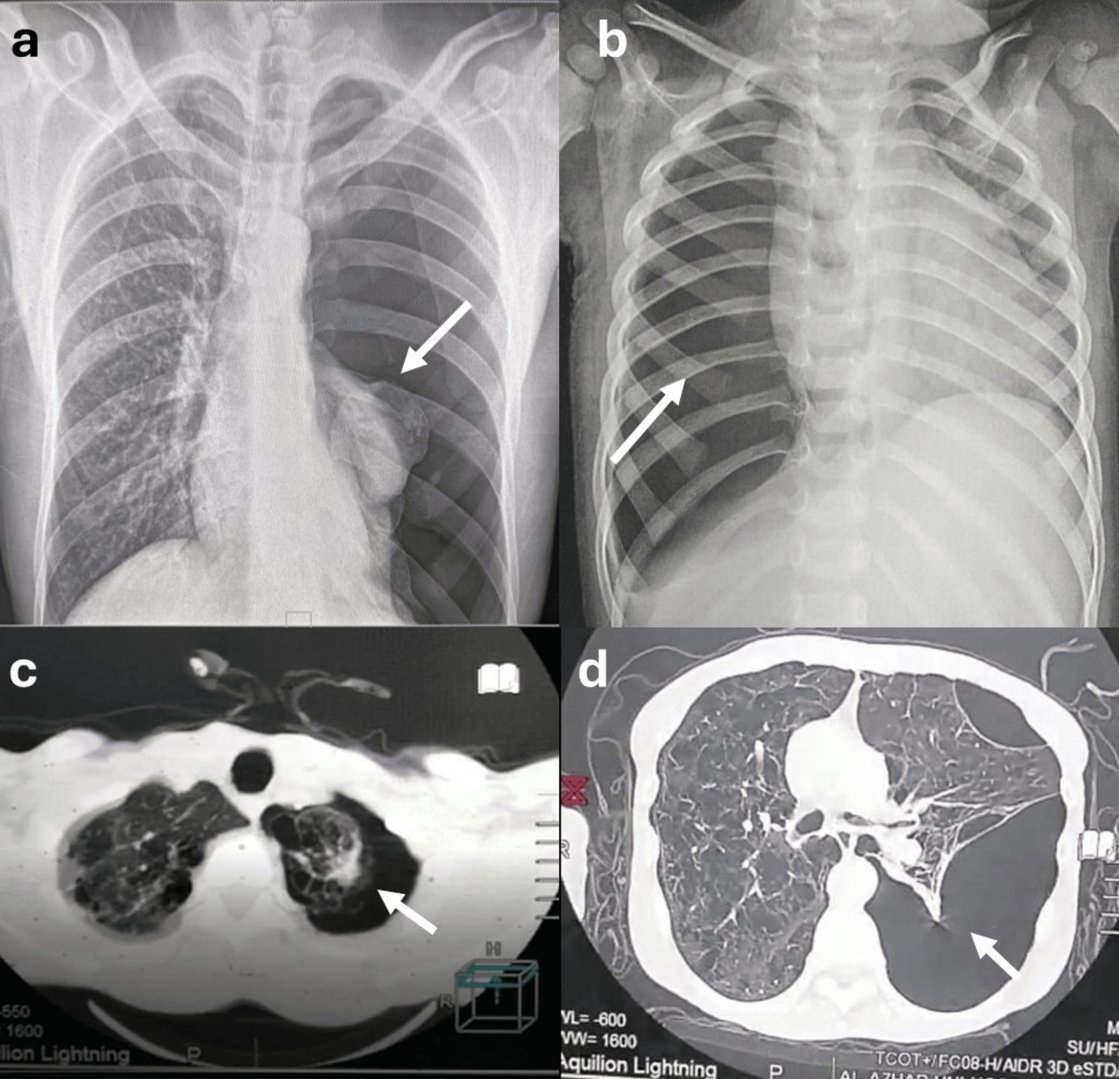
(a) X-ray of the chest (arrow indicates left side tension primary spontaneous pneumothorax); (b) X-ray of the chest (arrow indicates right side tension primary spontaneous pneumothorax); (c and d) CT of the chest (arrows indicate left side secondary spontaneous pneumothorax).
